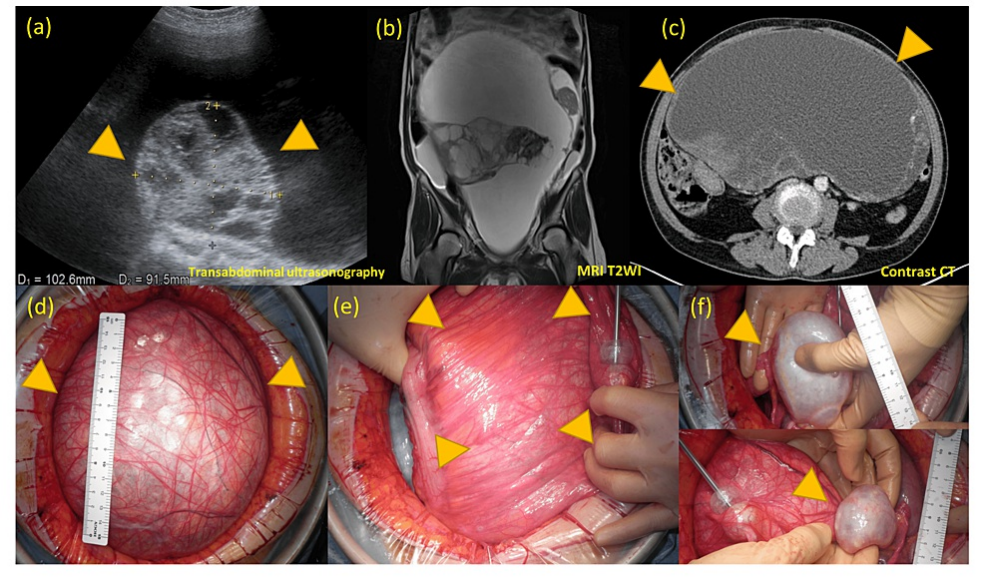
Pre-operative images of the computed tomography and laparotomy findings. (a, b, c) A gigantic multilocular cystic mass with a maximum diameter of 32 cm containing a solid mass. (d, e, f) The huge mass originated from the retroperitoneum; the bilateral ovaries were normal.
